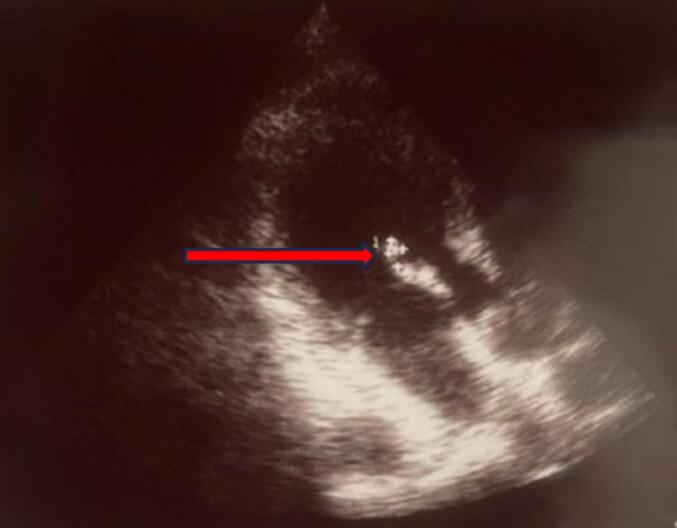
Echocardiography shows thickened anterior mitral valve leaflet with a vegetation of 7 × 4 mm with moderate eccentric mitral regurgitation (red arrow).
